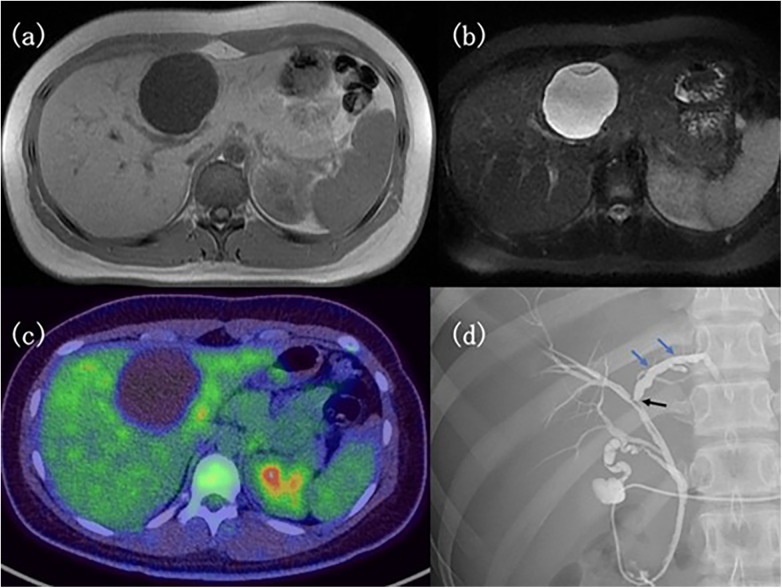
Advanced imaging findings of a hepatic cystic lesion. (a) T1-weighted magnetic resonance image showing a hypointense cystic lesion with internal septations. (b) T2-weighted magnetic resonance image showing a hyperintense cystic lesion. (c) FDG-PET/CT demonstrating no fluorodeoxyglucose uptake within the lesion. (d) Endoscopic retrograde cholangiopancreatography showing mild compression and dilatation of the left hepatic and anterior segment bile ducts (arrows). Reproduced from Moriuchi et al., Cureus (41), licensed under the Creative Commons Attribution–NonCommercial 4.0 (CC BY-NC 4.0) license, Cureus.
